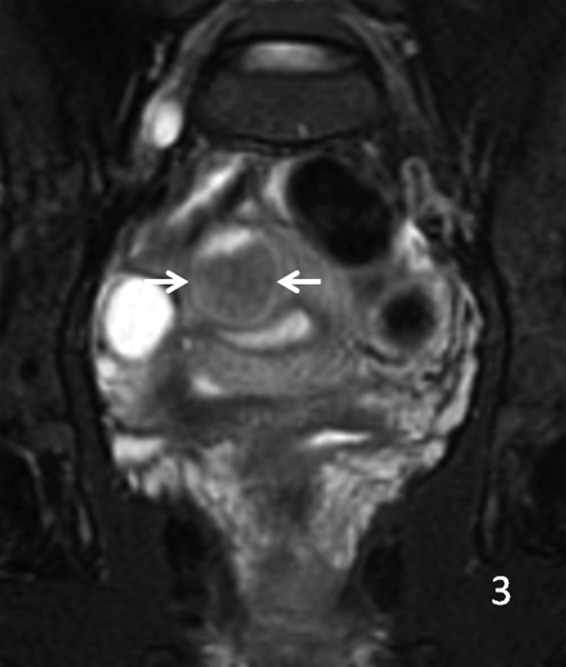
Coronal T2 fat saturation MRI image, with arrows showing signal strength in the mass.
